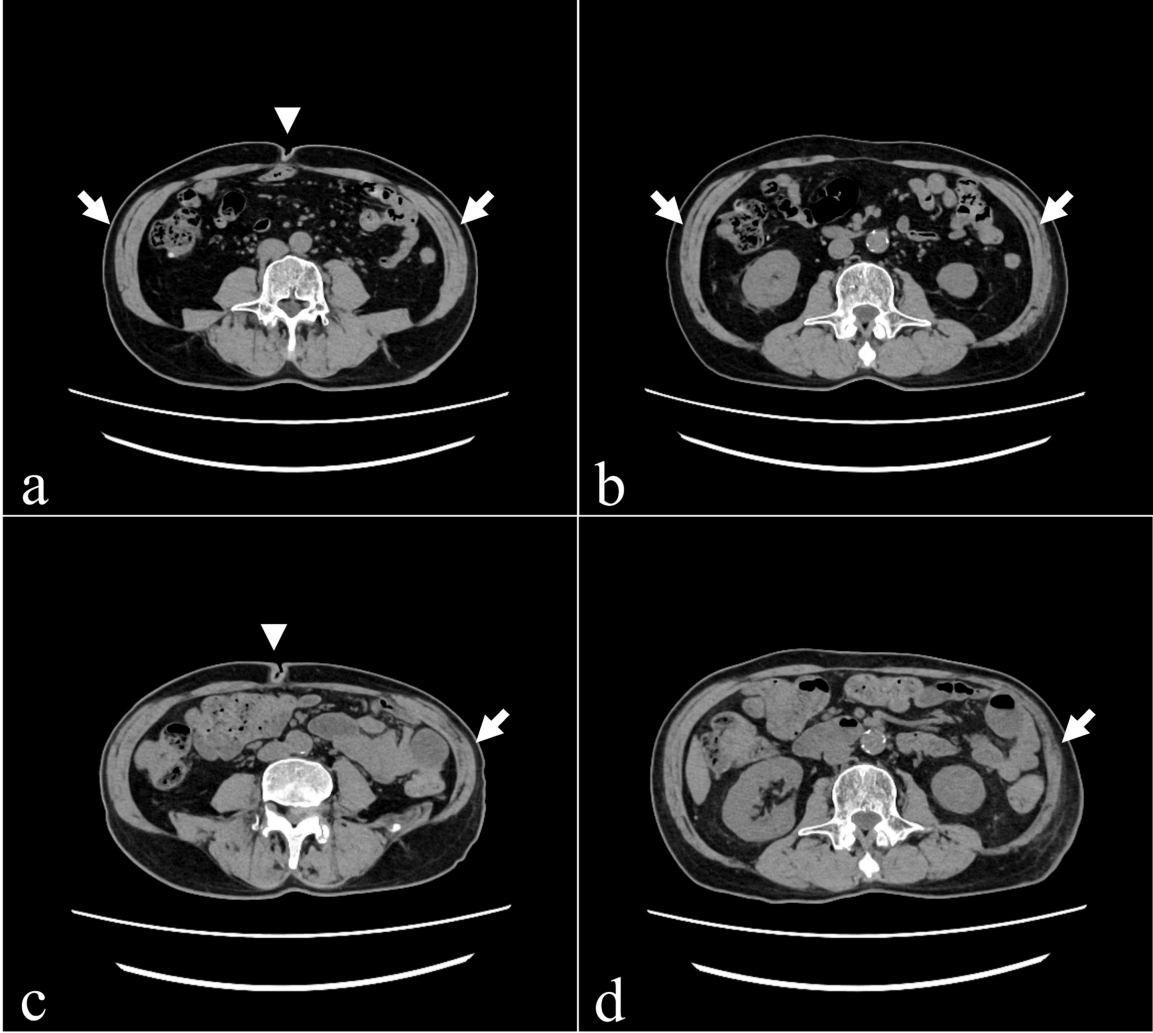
Comparison of abdominal CT images before and after irradiation (a) The initial abdominal CT image obtained at the start of radiation therapy reveals no apparent asymmetry between the left and right oblique muscles at the level of the umbilicus (arrows), with the umbilicus positioned near the midline but slightly shifted to the right (arrowhead). (b) At the level of the lower pole of the kidney, a similar observation is noted, with no discernible difference in the oblique abdominal muscles bilaterally (arrows). (c) In contrast, the abdominal CT image taken post-irradiation demonstrates thinning of the left oblique abdominal muscle at the level of the umbilicus (arrow). Additionally, a slight displacement of the umbilical region to the right is observed, indicating a shift toward the unaffected side (arrowhead). (d) At the level of the lower pole of the kidney, further evidence of thinning in the left oblique abdominal muscle is also noted (arrow).
